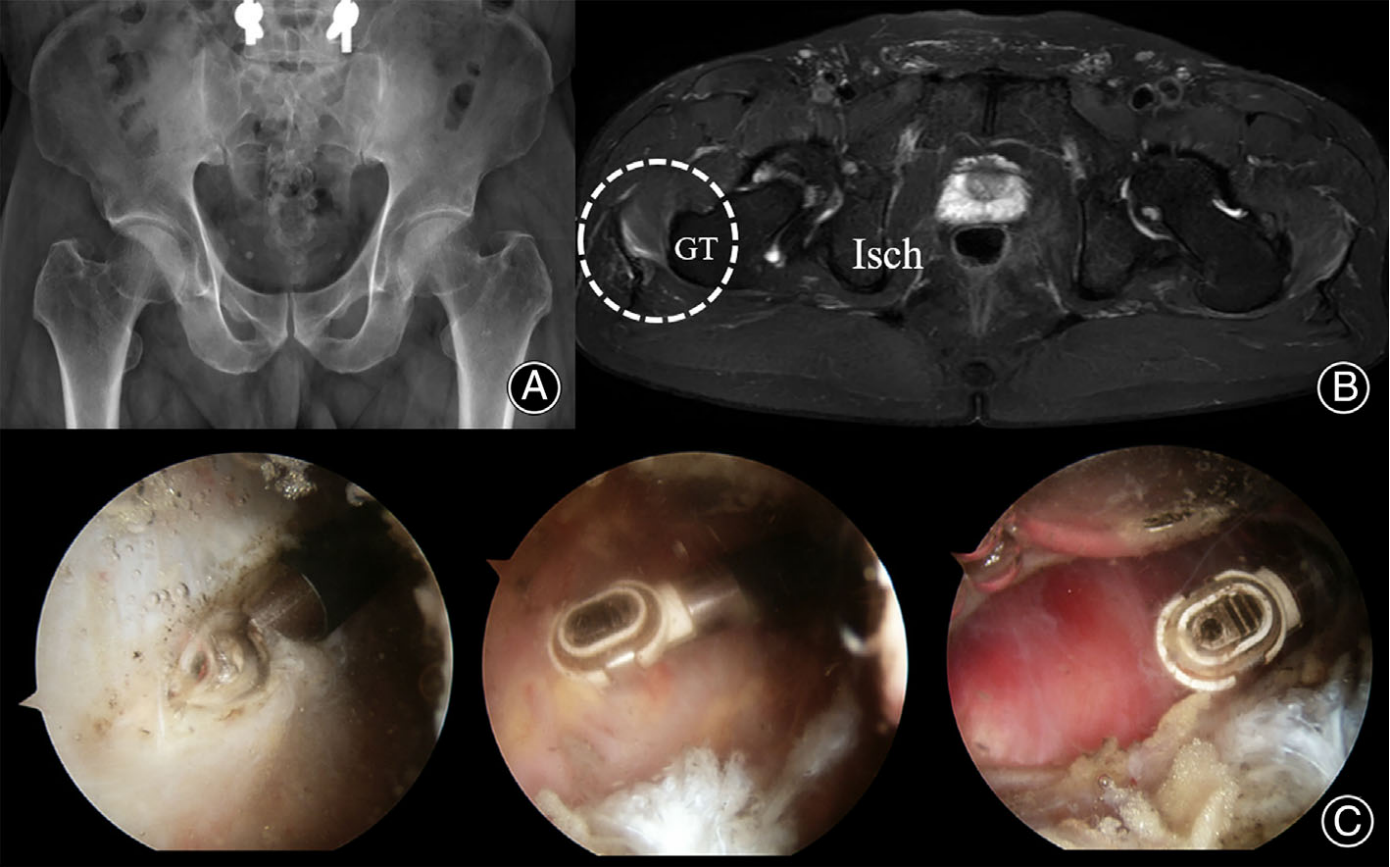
The pre‐ / post‐operative images and arthroscopic finding of 41‐year‐old male patient who underwent arthroscopic iliotibial band release. The simple hip X‐ray covers the symptomatic region (A), the T_2_‐weighted magnetic resonance image show the signal change between iliotibial band and GT on right hip (B, circle), and arthroscopic images show maximal arthroscopic resection with GT bursectomy (C), but the mild snapping persisted intra‐operatively. GT, greater trochanter.
